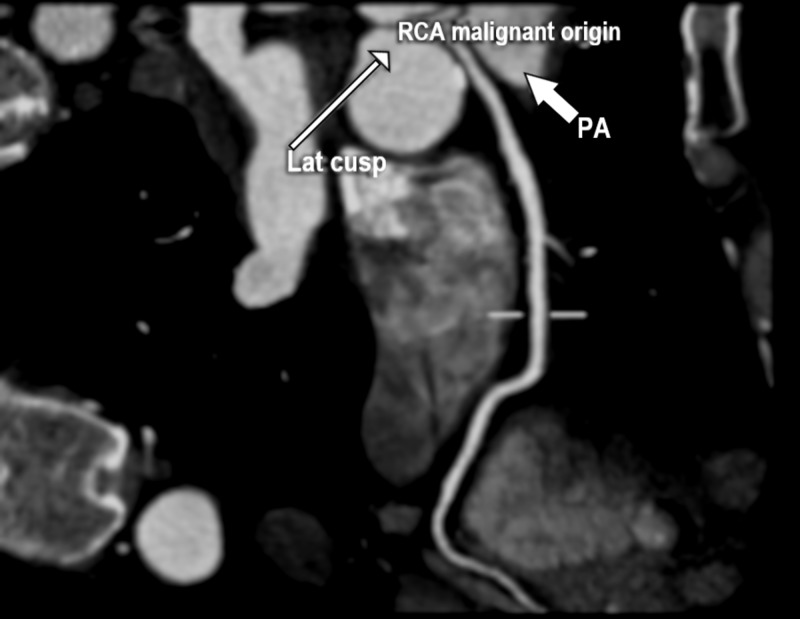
Computed tomography angiography, malignant course Malignant course of the right coronary artery between the aorta and the pulmonary trunk. Lat cusp=lateral cusp of aorta; PA=pulmonary artery.
